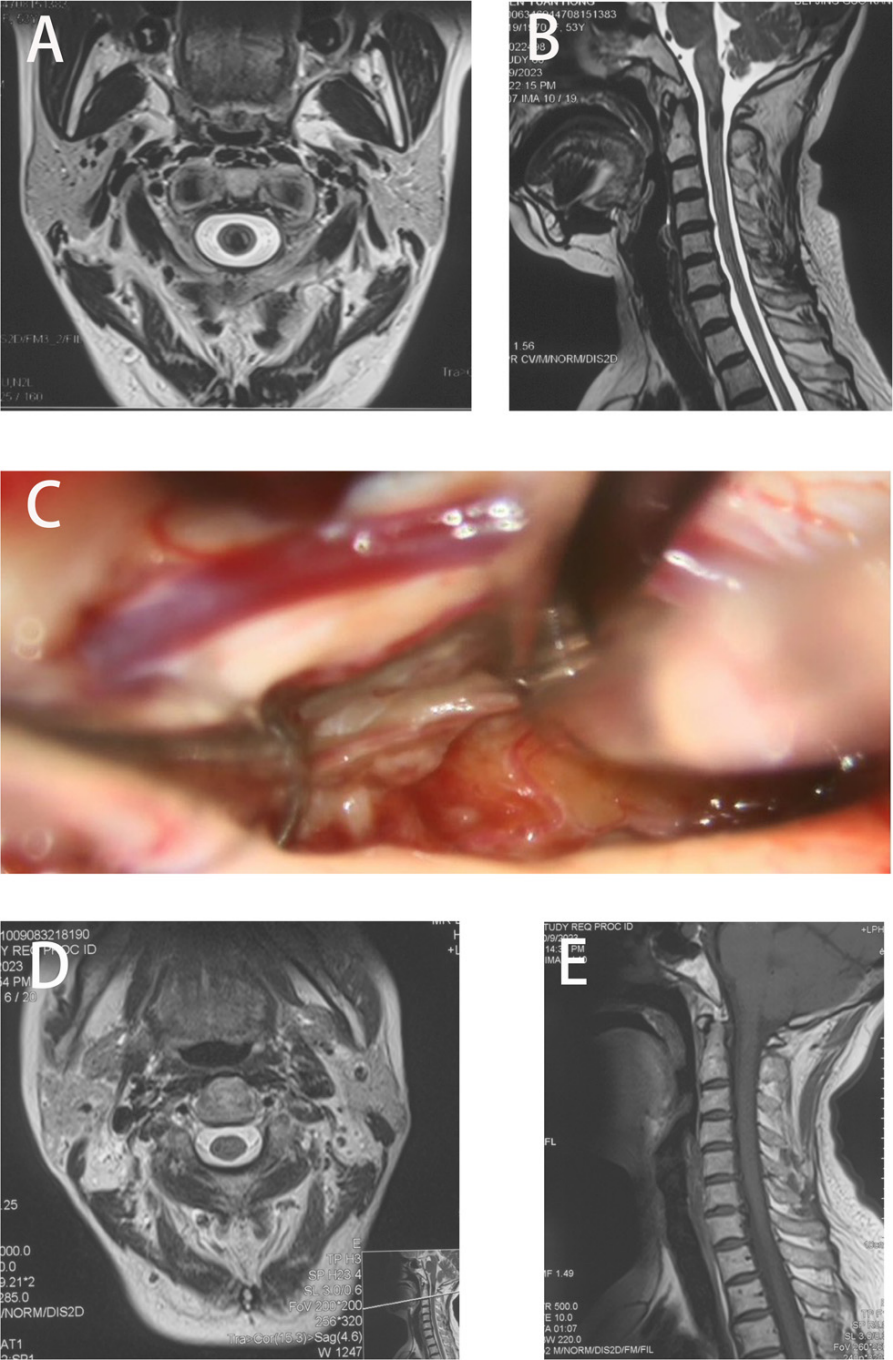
Preoperative MRI (a) and (b), and intraoperative findings (c) showed an SCM at C1–C2 level. Postoperative MRI (d) and (e).
